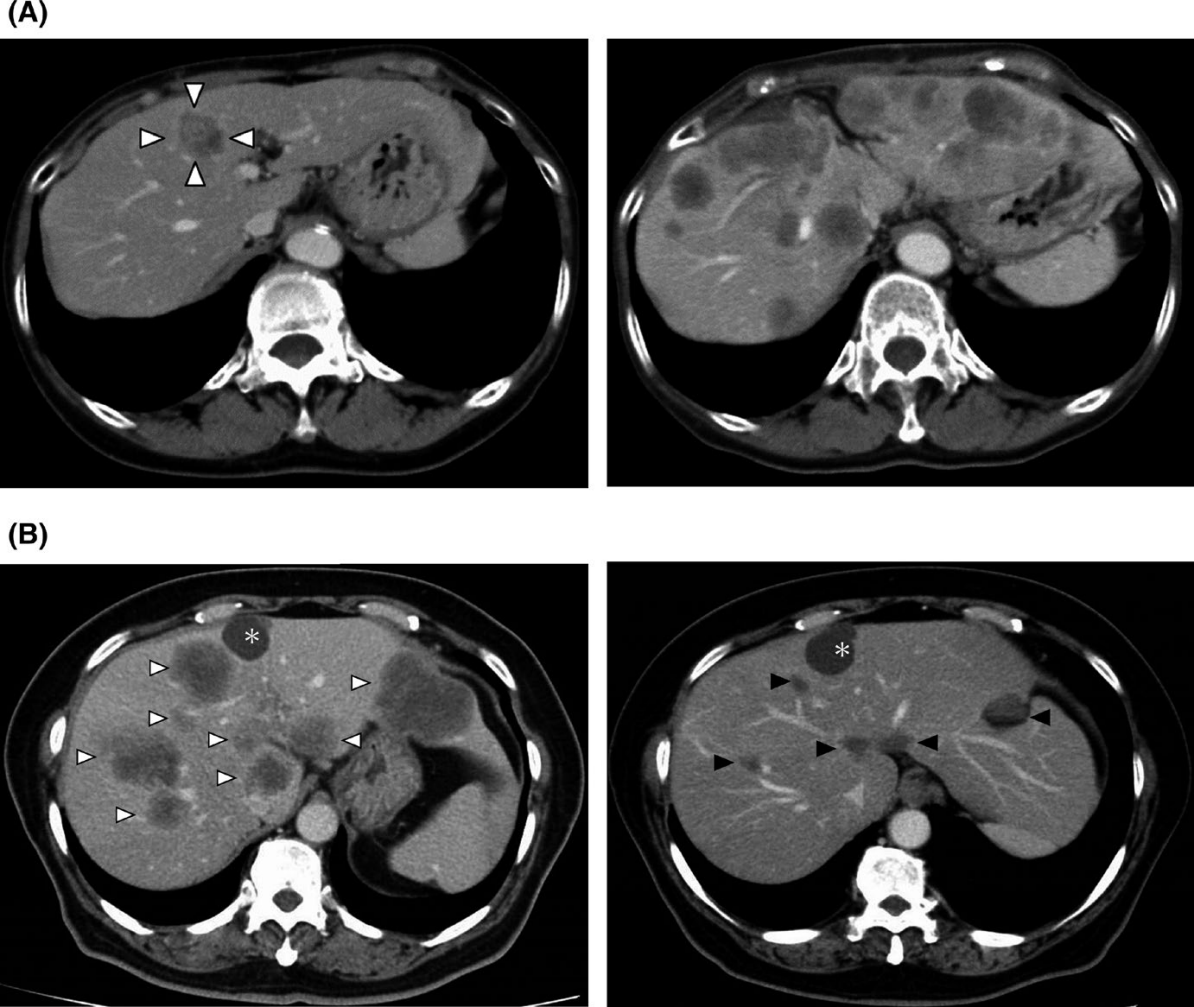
Computed tomography findings of representative patients with BRAF V600E mutation. A, A solitary resectable case. (left) A 71‐year‐old female patient who underwent hepatectomy for metachronous solitary CRLM (triangles). (right) However, the patient developed multiple liver metastases along with lung and peritoneal metastases 4.4 months after hepatectomy and died 6.8 months after surgery. B, An unresectable case. (left) A 61‐year‐old female patient developed metachronous multiple unresectable CRLM (white triangles) and peritoneal metastases along with a liver cyst (asterisk). (right) After receiving FOLFOXIRI plus bevacizumab for 9 months, the CRLM shrunk with good response (black triangles). Afterward, she received the BEACON CRC triplet regimen (encorafenib, binimetinib, and cetuximab) with other treatments and died 36.0 months after the start of the first‐line chemotherapy
